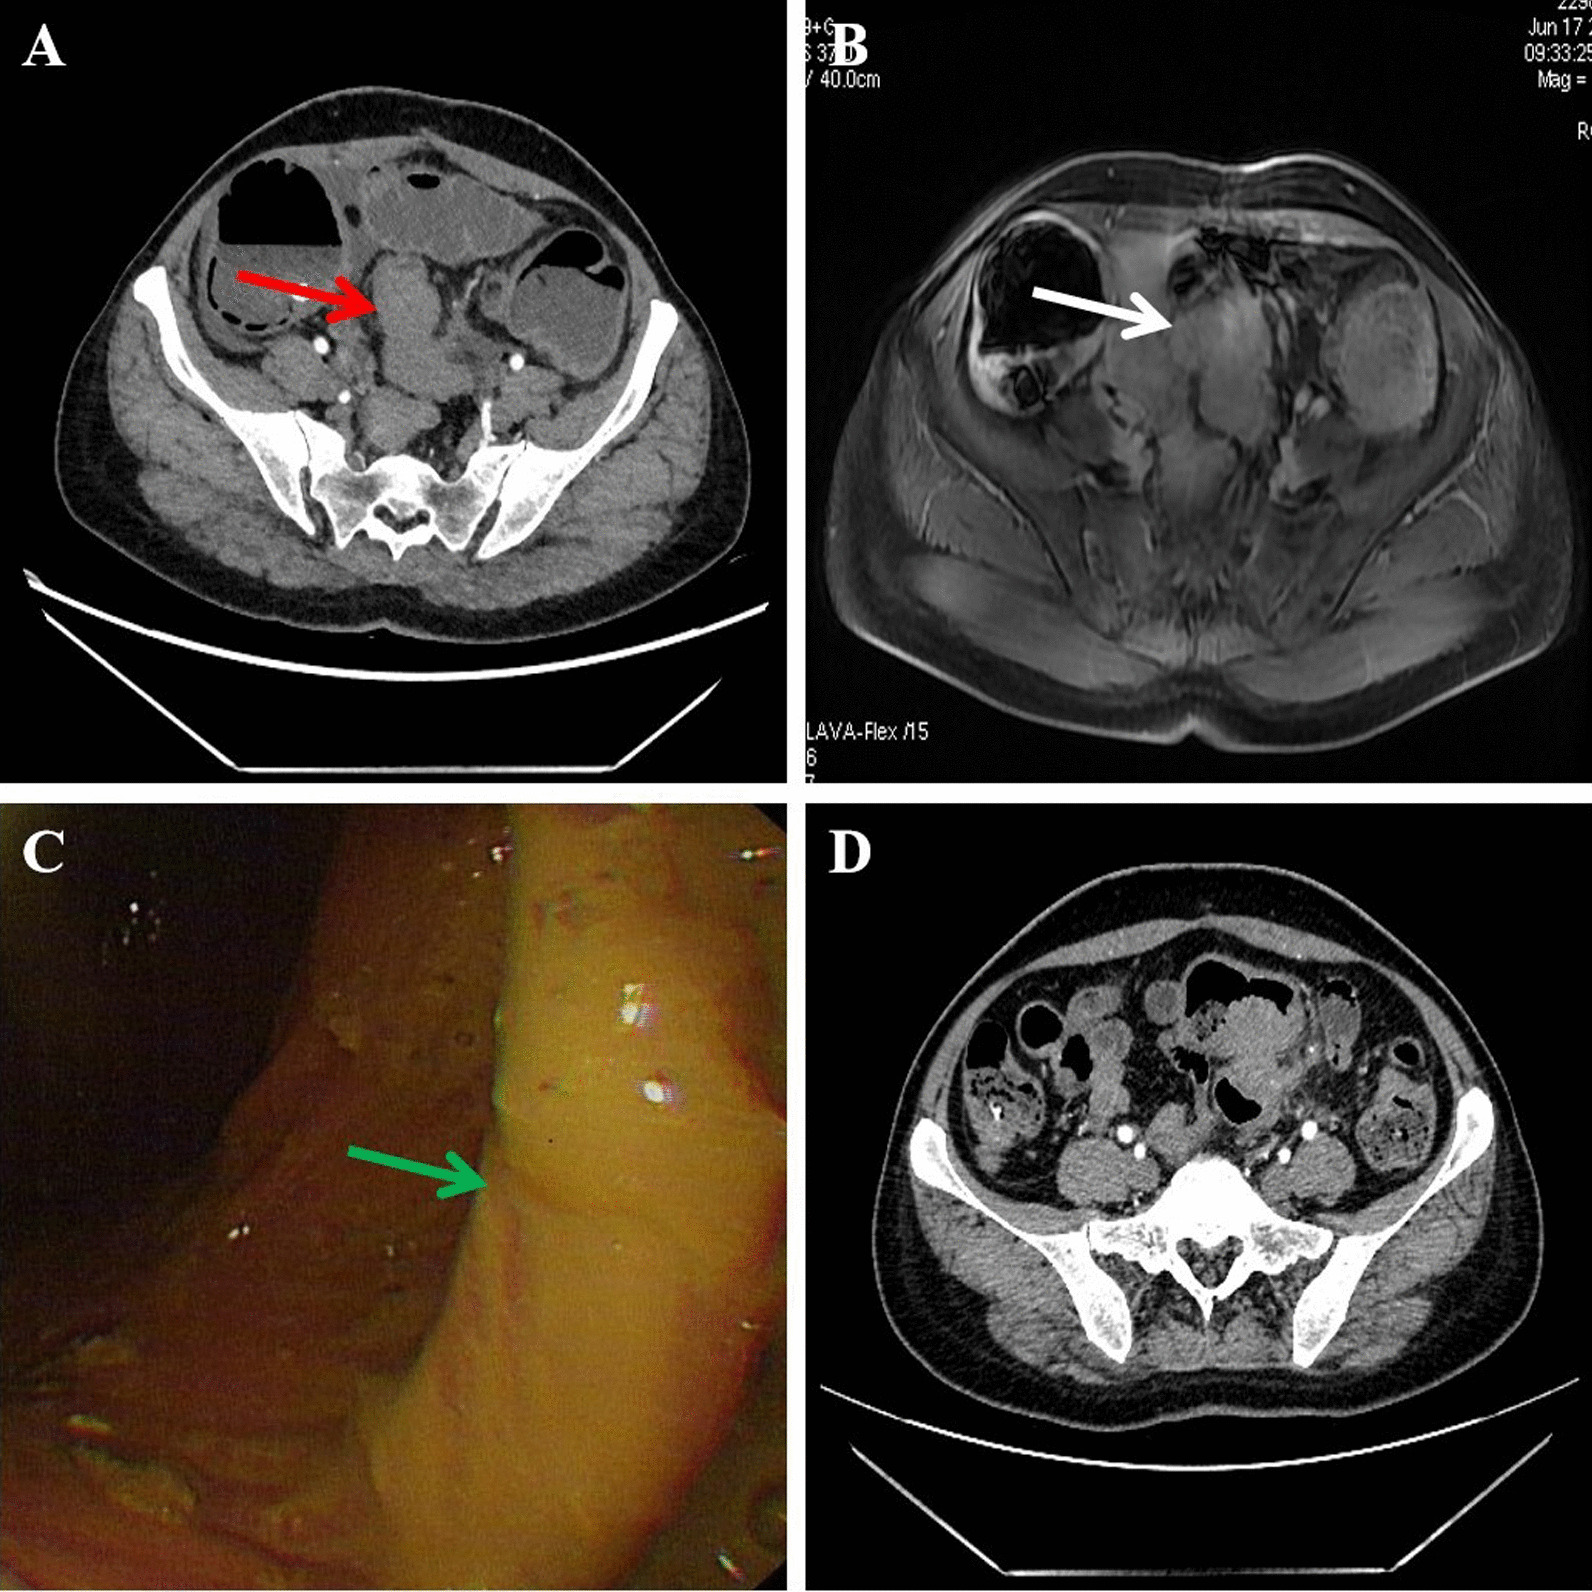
Preoperative examination of the patient. A Computed tomography (CT) revealed wall thickening from the upper rectum to sigmoid, accompanied by low intestinal obstruction (red arrow). B Magnetic resonance imaging (MRI) revealed neoplastic lesions of the sigmoid colon (white arrow). C Colonoscopy showed stenosing tumor formation in the upper rectum, the mucosal surface is smooth (green arrow). D After 7 days of colon decompression, computed tomography reexamination showed the dilatation of the colon was significantly less than before
